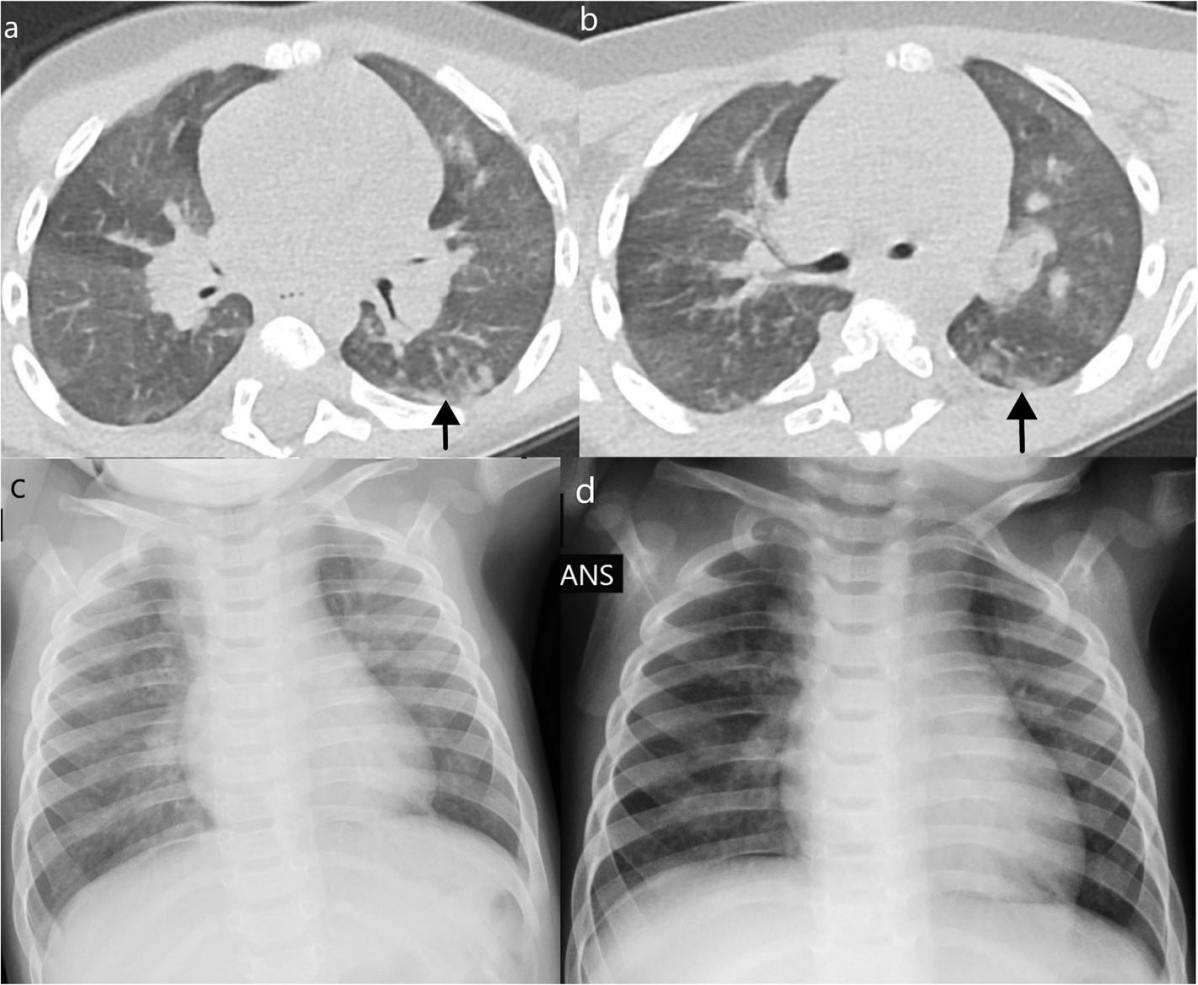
Indeterminate features in a 7-month-old infant. Chest CT on day 5 of symptoms (a, b) showed diffuse GGOs with no peripheral or central predominance. Bronchial wall thickening was also noted (not shown). This patient was coinfected with adenovirus and RSV which could partially be responsible for the mosaic attenuation pattern/small airway disease. Linear opacities in the posterior subpleural lung (arrows) are commonly seen in infants representing athelectasic bands due to hypoventilation of the decubitus. Chest X-ray from the same patient (c) shows diffuse reduced lung transparency bilaterally, with no predominant distribution. Opacities almost completely resolved 8 days later (d)
